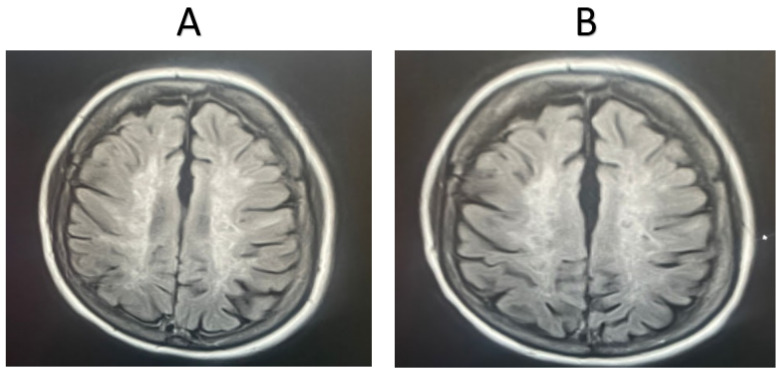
MRI examination of an MSW patient taking fingolimod. (A). The number of lesions before treatment = 13, with an average lesion size (cm) of 0.36 ± 0.21, (B). The number of lesions after treatment = 6, with an average lesion size (cm) of 0.17 ± 0.24. The number and extent of the lesions were reduced after one year of fingolimod treatment.
